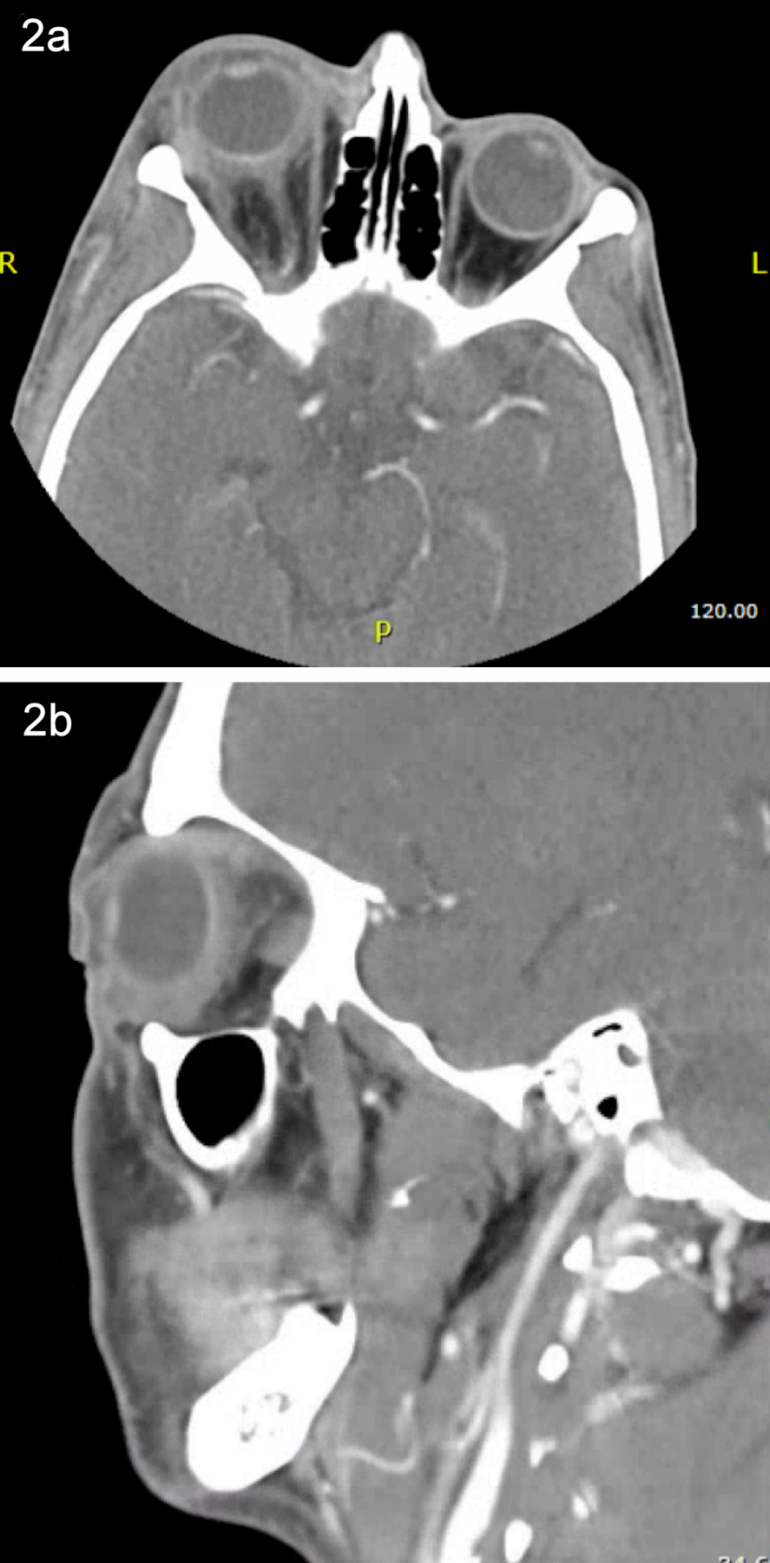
Axial (a) and sagittal (b) computed tomography (CT) post-contrast images showing proptosis of the right eye with orbital fat stranding focused primarily around the globe. There is no evidence of sinus disease or subperiosteal abscess.
